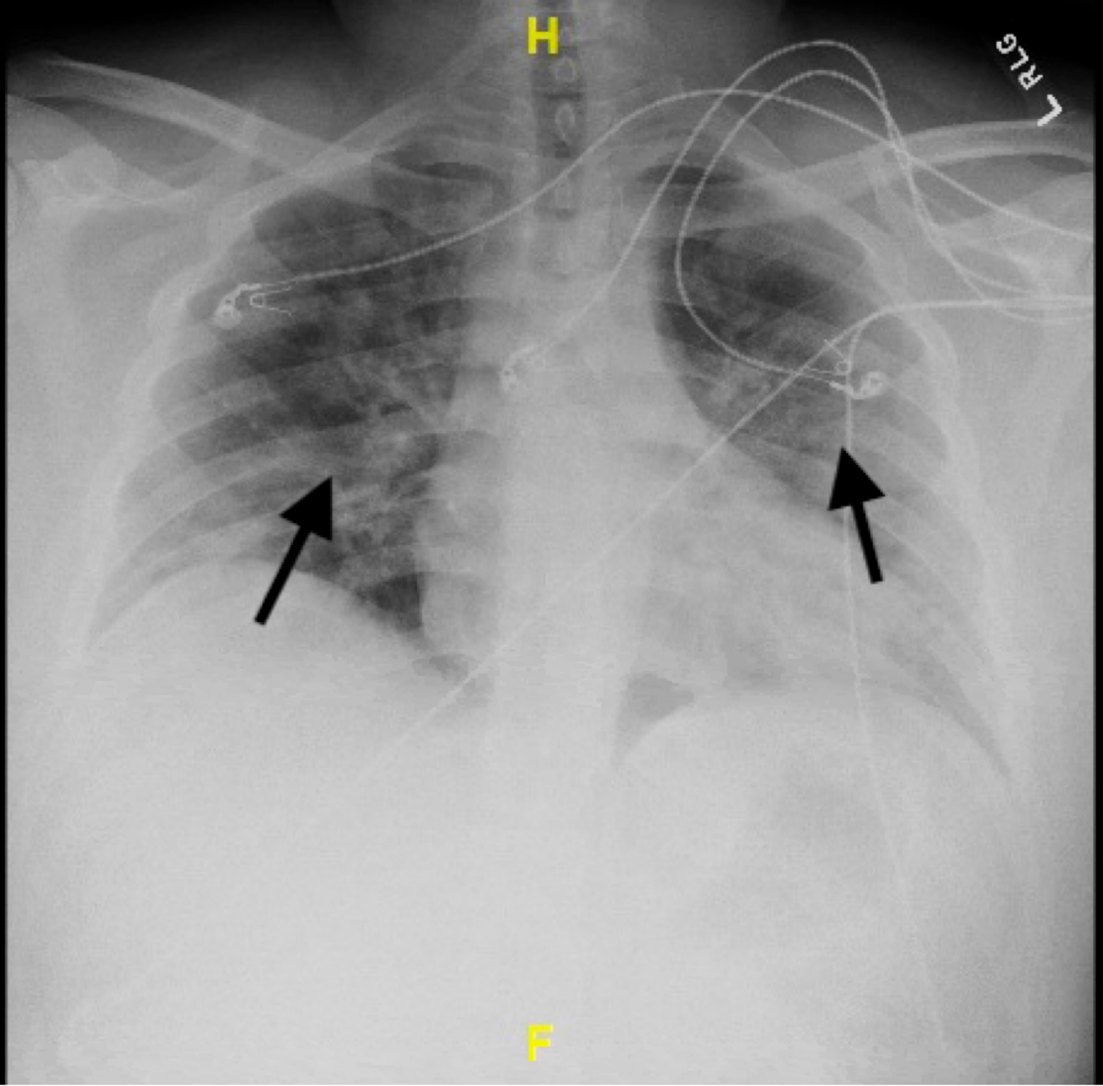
One view chest X-ray on presentation revealing bilateral patchy infiltrates.
